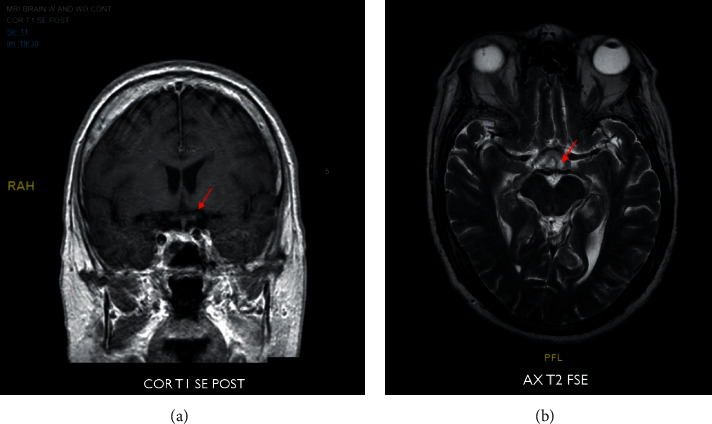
MRI of the brain with and without contrast—small region of focal water restriction at the left mammillary body consistent with a subtle infarct in this area.
